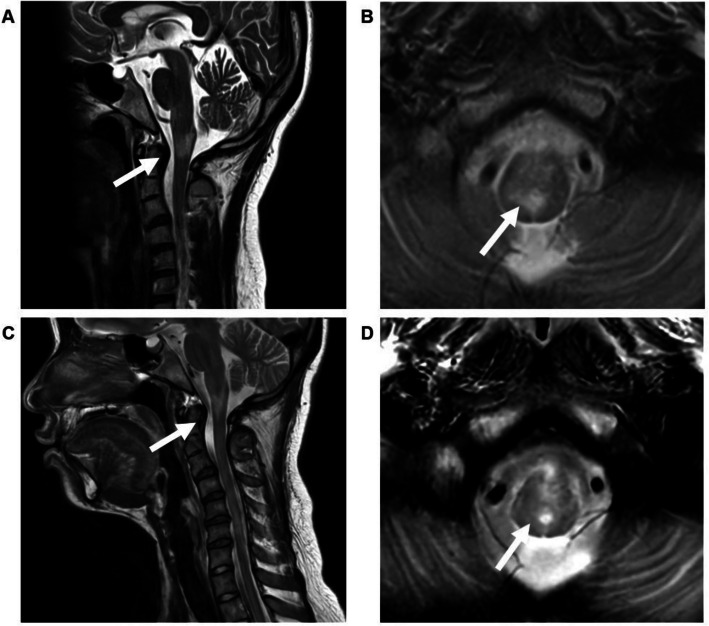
Magnetic resonance imaging (MRI) findings. Sagittal (A) and axial (B) T2-weighted images at 35 weeks of gestation show hyperintense lesions from the lower medulla to the upper thoracic spinal cord. The prepartum hyperintense lesions show improvement, and no new abnormalities are identified in sagittal (C) and axial (D) T2-weighted magnetic resonance images obtained after delivery. Arrows indicate the affected areas
